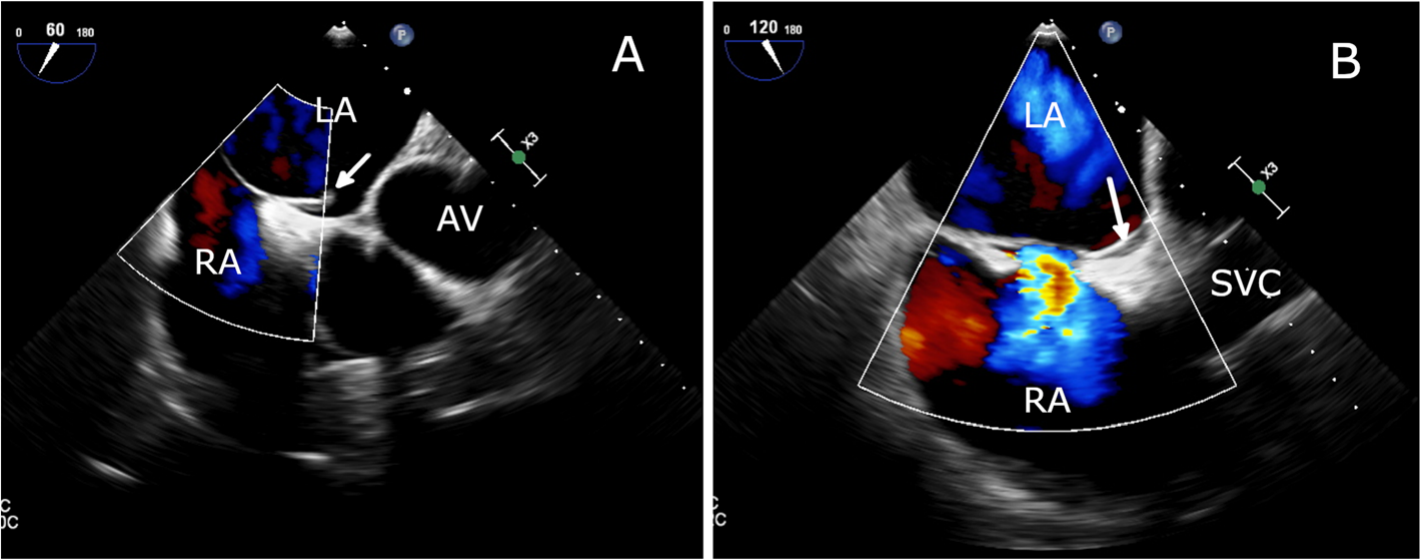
Left atrial septal pouch thrombus seen by color Doppler imaging during TEE at 60° [a] and 120° [b] and simultaneously excluding any probable atrial septal defect
